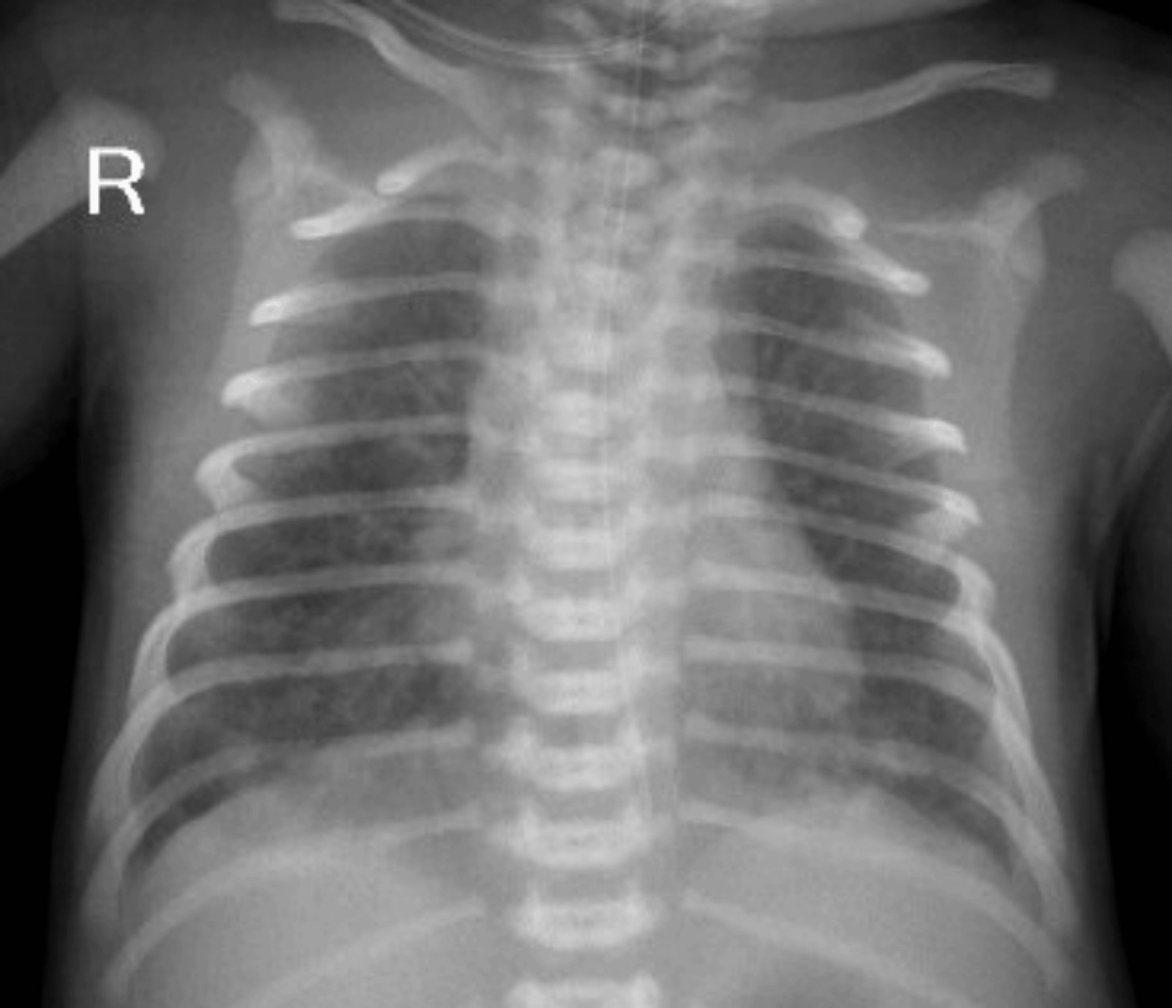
Chest radiograph obtained in the early neonatal period Anteroposterior chest radiograph obtained within the first 24 hours of life demonstrating bilateral patchy pulmonary opacities, more prominent in the perihilar and lower lung zones consistent with parenchymal disease. These findings were interpreted in the clinical context as suggestive of early-onset congenital pneumonia.
